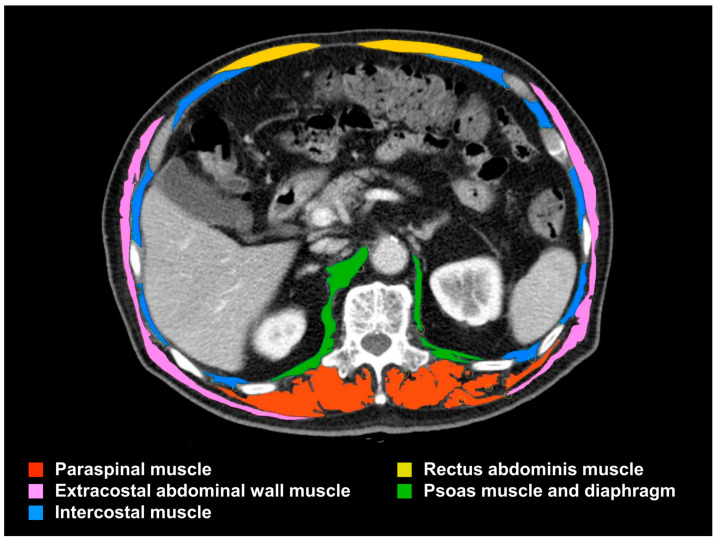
CT-based assessment of L1 skeletal muscle mass. The axial skeletal muscle area at the L1 level comprises five major components: paraspinal muscle, extracostal abdominal wall muscle, intercostal muscle, rectus abdominis muscle, and psoas muscle and diaphragm. CT, computed tomography; L1, first lumbar vertebra.
